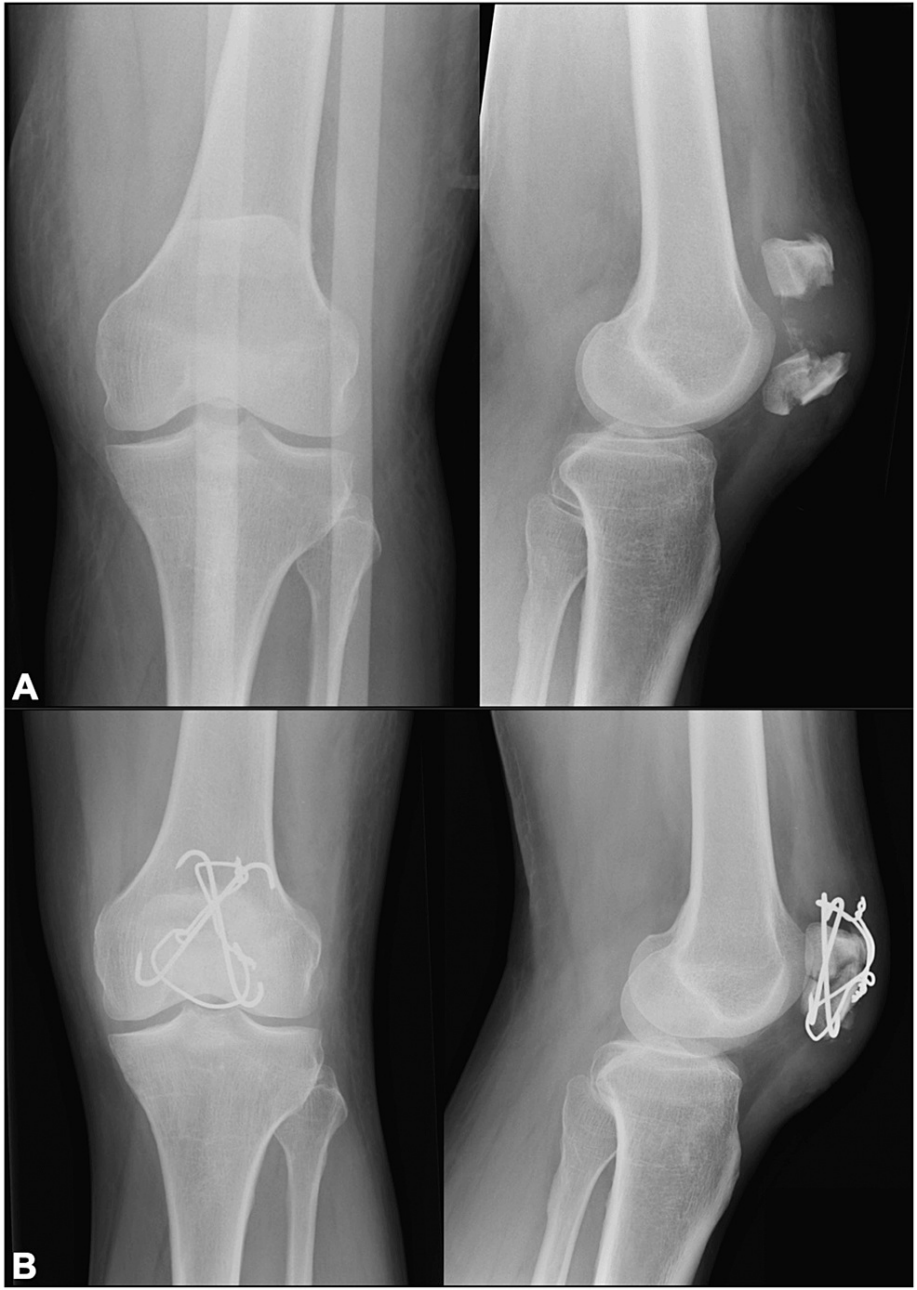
Preoperative (A) and postoperative (B) anteroposterior and lateral X-rays of a patellar fracture managed with the modified crossed pin tension band technique.
